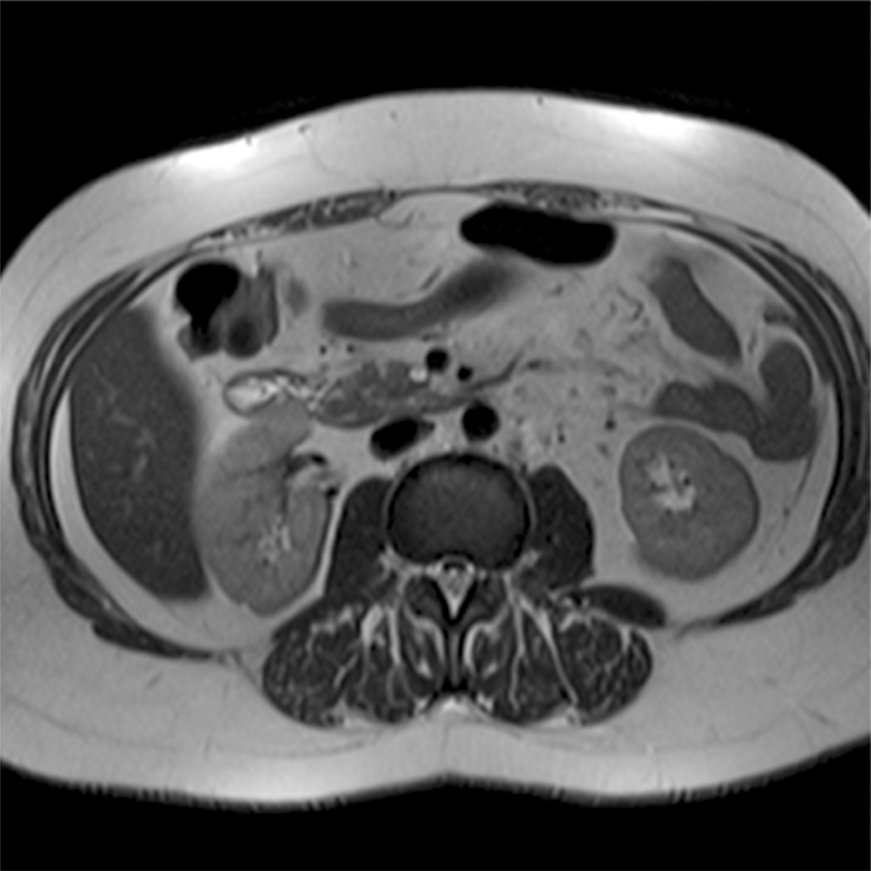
Abdominal MRI (T2-weighted sequence, axial slice), showing a small cyst with thin septum located in the uncinate process of the pancreas.
